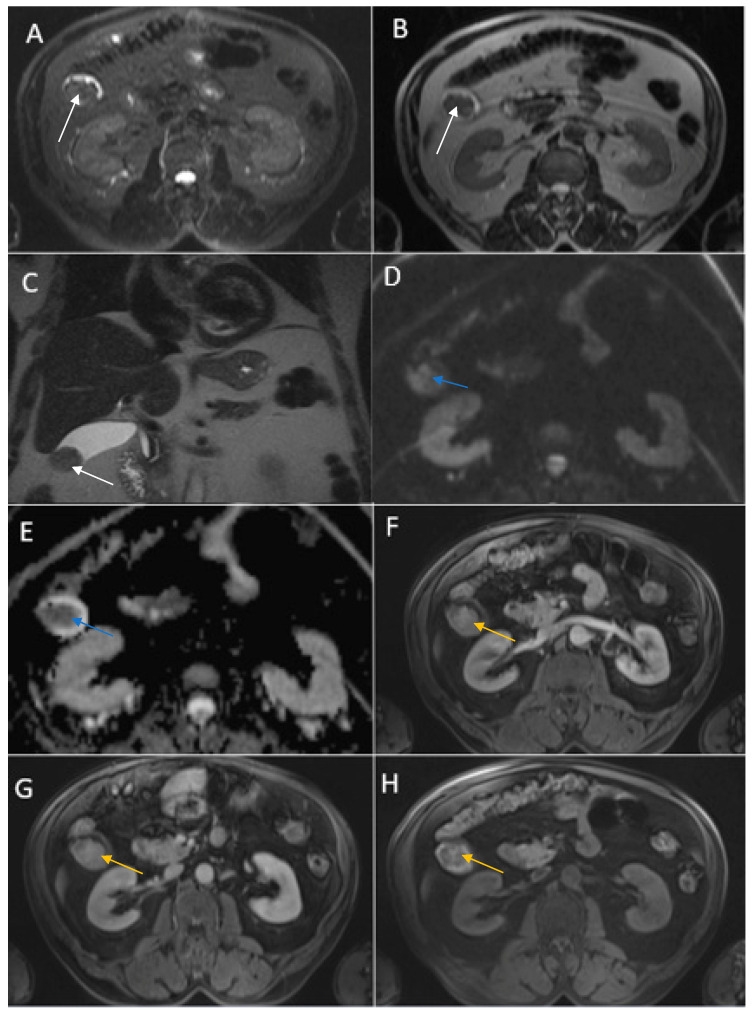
Abdominal MRI. (A) Axial T2-weighted FS showing markedly hypointense pseudonodular tumoral gallbladder lesion (white arrow). (B) Axial T2-weighted showing hypointense gallbladder lesion (white arrow). (C) Coronal T2-HASTE hypointense gallbladder lesion (white arrow). (D) Diffusion-weighted imaging (DWI B800) showing bright hypersignal of the lesion (blue arrow). (E) Restricted diffusion (blue arrow) on apparent diffusion coefficient (ADC) map. (F,G) Axial contrast-enhanced T1-weighted image showing a strongly enhancing polypoid pseudonodular mass in the gallbladder fundus. (H) Axial T1-weighted image FS showing hyperintense nodular lesion component (yellow arrows), highly suggestive of melanin.
